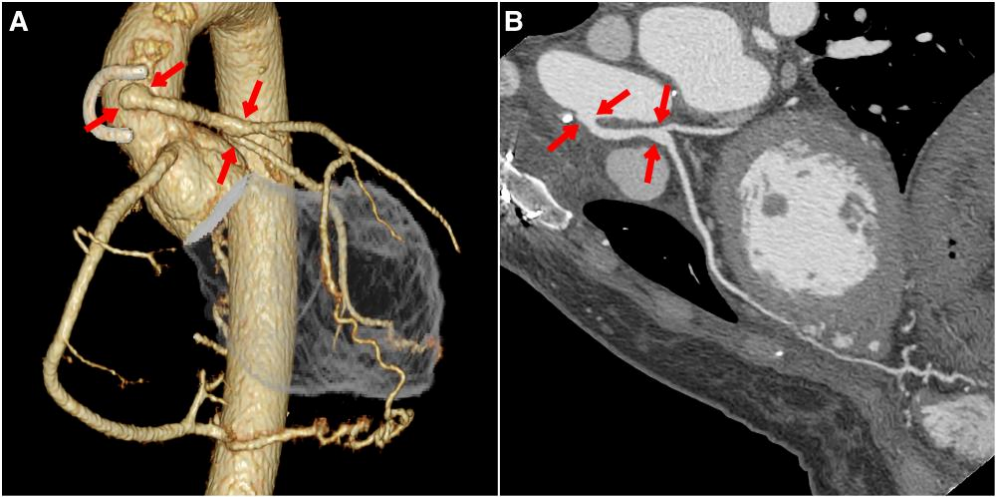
Post-operative coronary computed tomography images of volume rendering (*A*) and multiplanar reconstruction (*B*). The harvested external iliac artery is anastomosed as a free graft from the aorta to the proximal portion of the left coronary artery. The anastomotic sites are indicated by arrows.
